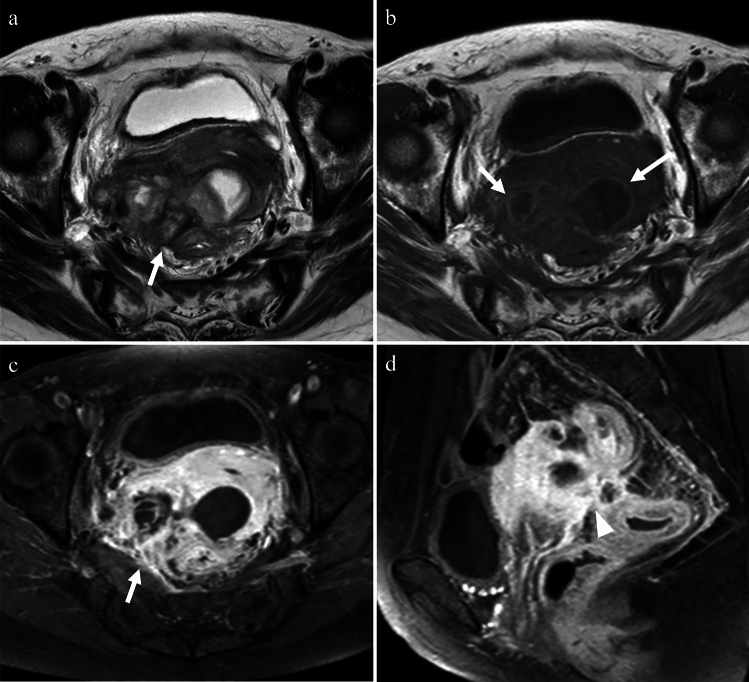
Woman in her 60 s with a history of intrauterine device use who presented with genital bleeding and tested positive for Actinomycosis. a A solid cystic mass, adhered extensively to the uterus, is observed with an indistinct border. The cystic contents exhibit heterogeneity on T2-weighted image. b The presence of rim-like high signal intensity at the cyst margins (arrows) on T1-weighted image indicates the presence of abscesses. c The solid component demonstrates strong enhancement (arrow) on fat-saturated contrast-enhanced T1-weighted image (FS-CE-T1WI). d Infiltration into the rectum (arrowhead) is evident on sagittal FS-CE-T1WI
